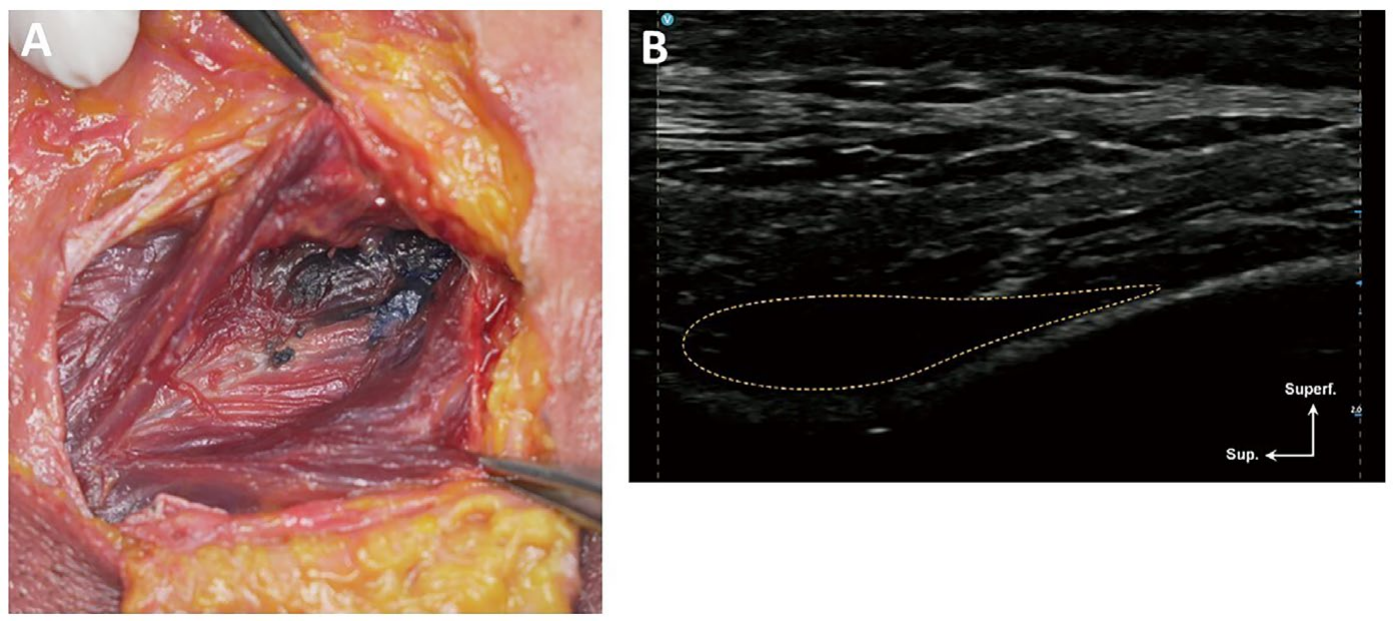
A demonstration of filler placement following the deep plane injection. technique in the temple (72‐year‐old Korean male). (A) Cadaver dissection. (B) Cadaver ultrasound.
